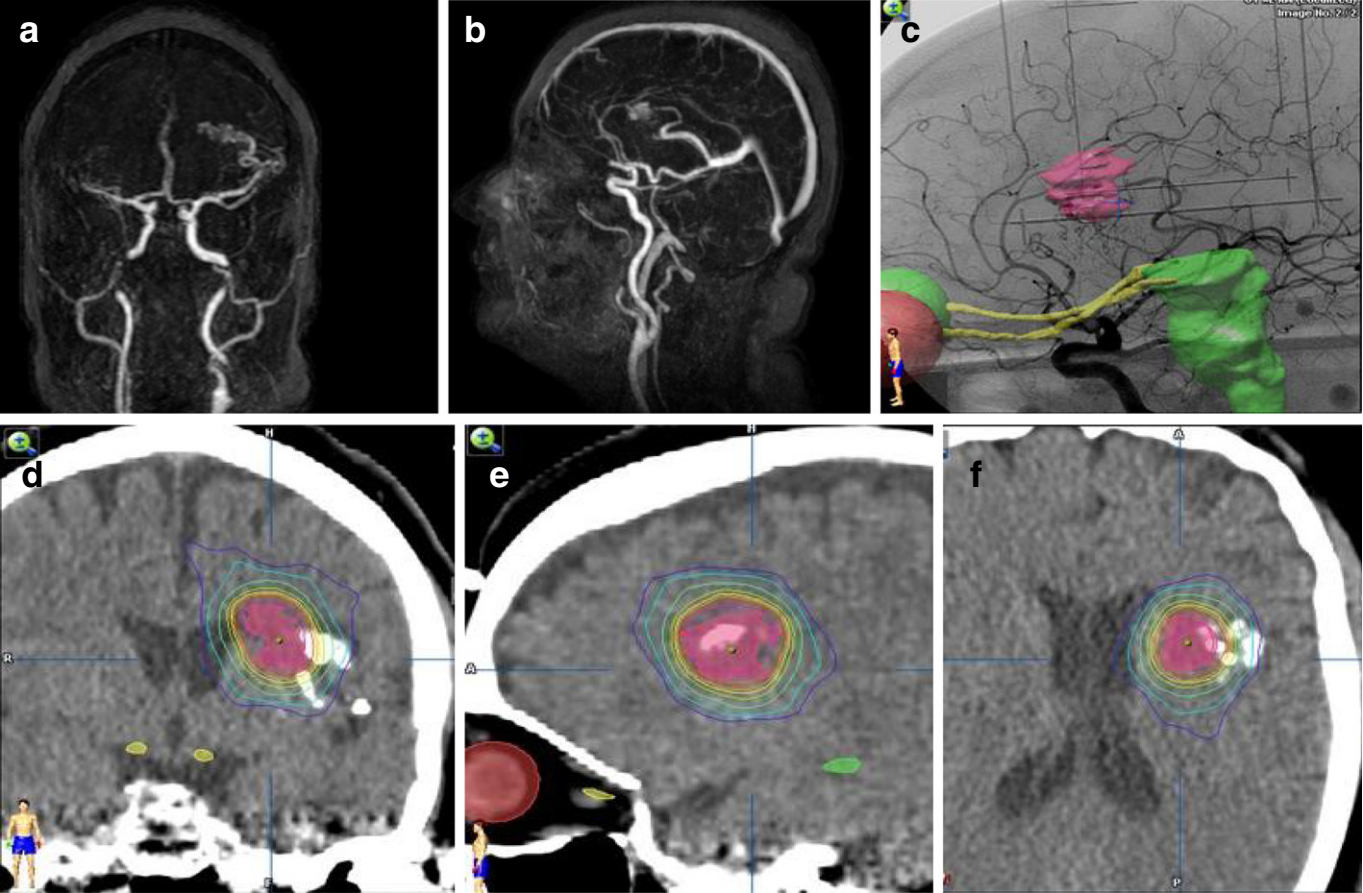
Incidental left frontal cerebral arteriovenous malformation in a 65 year-old woman after 3 partial embolizations (patient #1). Maximum intensity projections of pre-operative dynamic 4D-MR angiography (a: frontal view, b: saggital view), treatment planning (c: fused DSA and planning target volume (PTV), d-f: fused CT with PTV and isodose lines).
